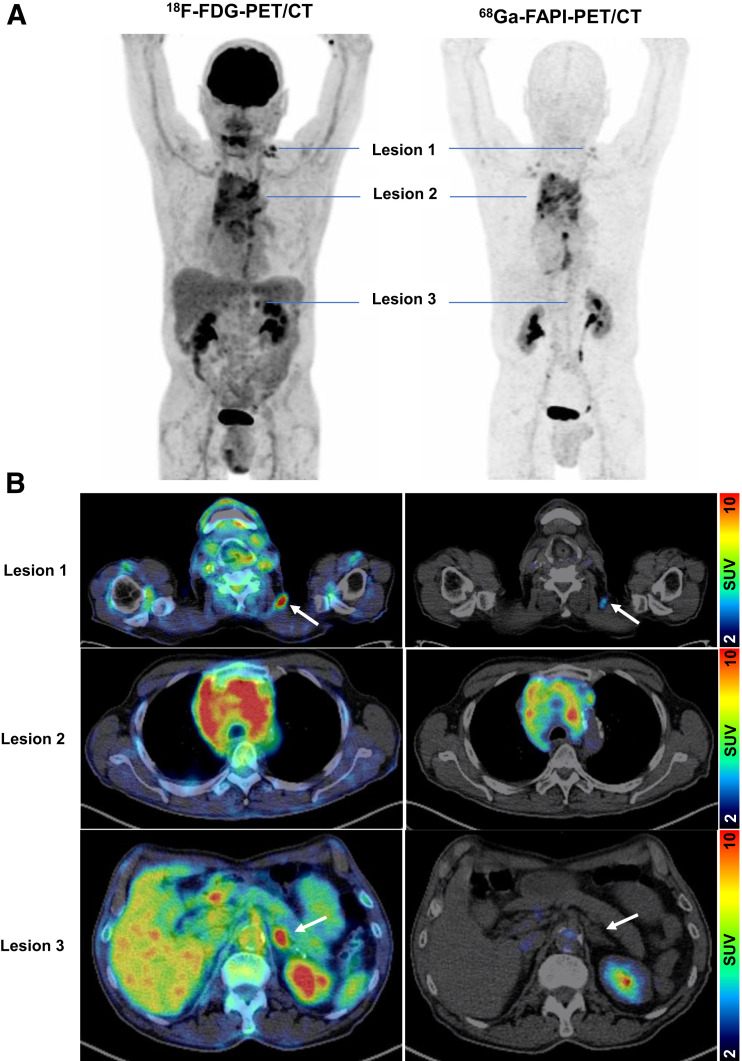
Example images of 78-y-old man with primary non–small cell lung cancer. (A) Maximum-intensity projection of ^18^F-FDG PET and ^68^Ga-FAPI PET. (B) Axial ^18^F-FDG PET/CT and ^68^Ga-FAPI PET/CT of suggestive lesions (arrows: lesion 1, cervical lymph node metastasis; lesion 2, non–small cell lung cancer with involvement of mediastinal lymph nodes; lesion 3, left adrenal mass). Although lesions 1 and 2 were clearly detectable by both PET scans, lesion 3 was ^18^F-FDG–avid but not confirmed by ^68^Ga-FAPI PET.
